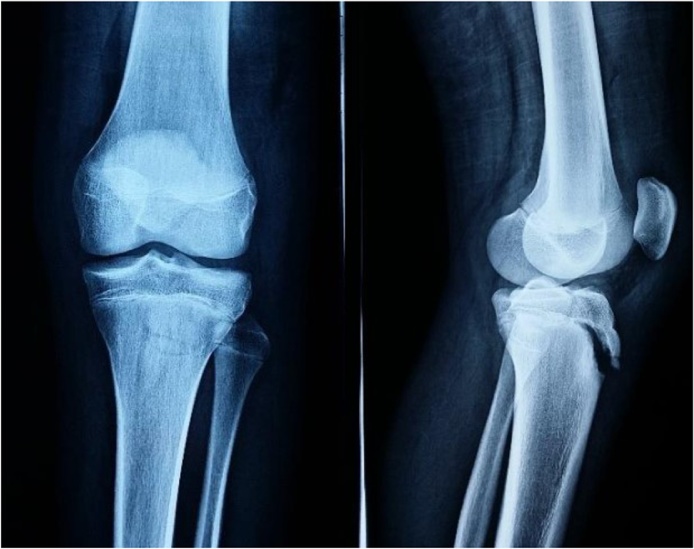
knee X-rays showing a displaced avulsion fracture of the tibial tuberosity.
